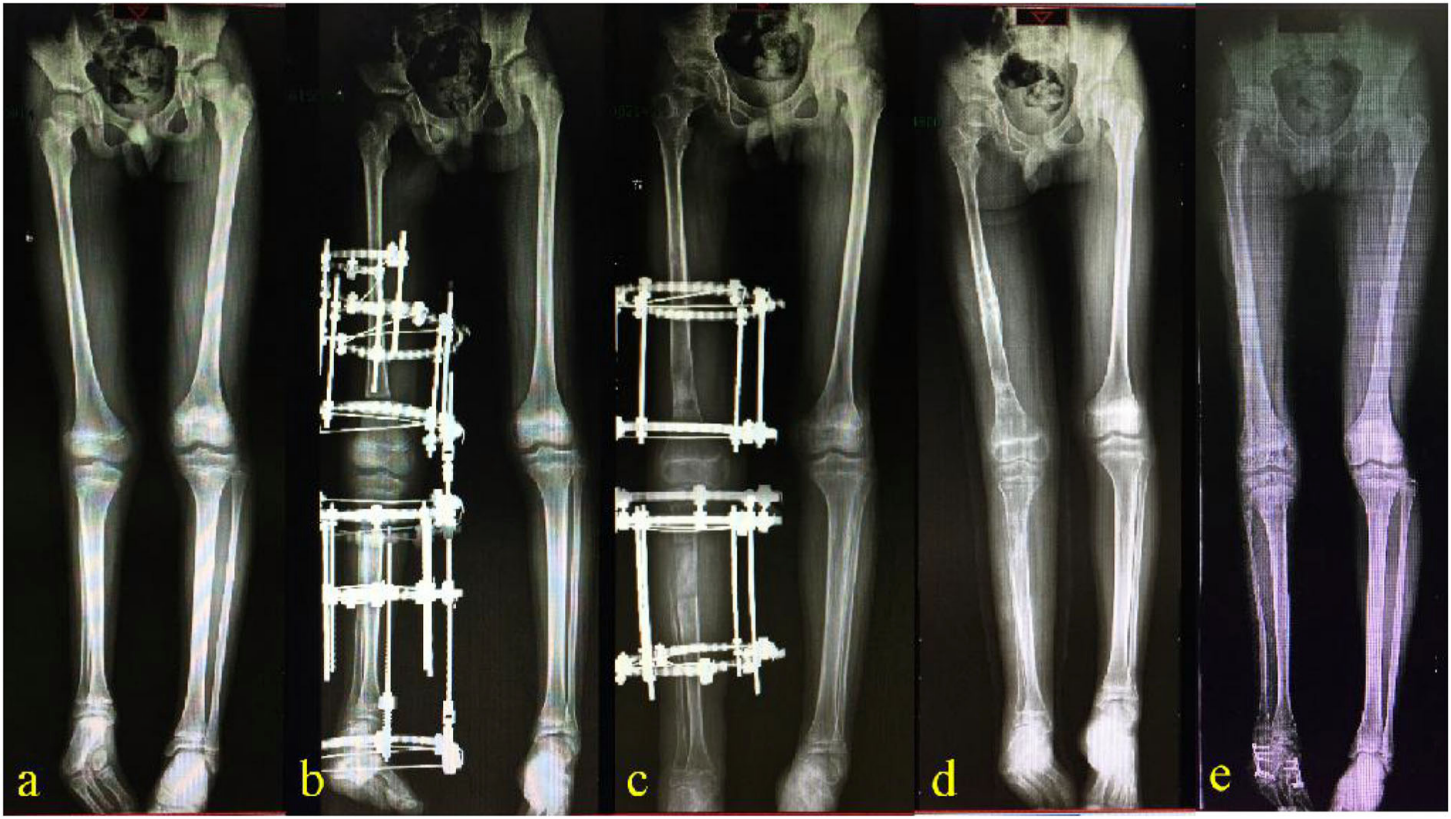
Anteroposterior X-ray view. (a) An 11.5-year-old boy showed congenital fibular deficiency with a total 8.8 cm shortening of the right femur and tibia, genu valgus, and was missing lateral feet in an equinovalgus position. (b) A double Ilizarov frame, including a femoral segment and a tibial segment, were connected with two straight/flexure device on the level of the knee joint. (c) The bony callus was observed almost consolidated 28 weeks after operation after lengthening, lasting for 5 weeks on the femur and 8 weeks on the tibia. (d) After 40 weeks of the operation, the double-Ilizarov frame was removed. (e) At a 3.5-year postoperative follow-up, the limb length discrepancy was 1.5 cm.
